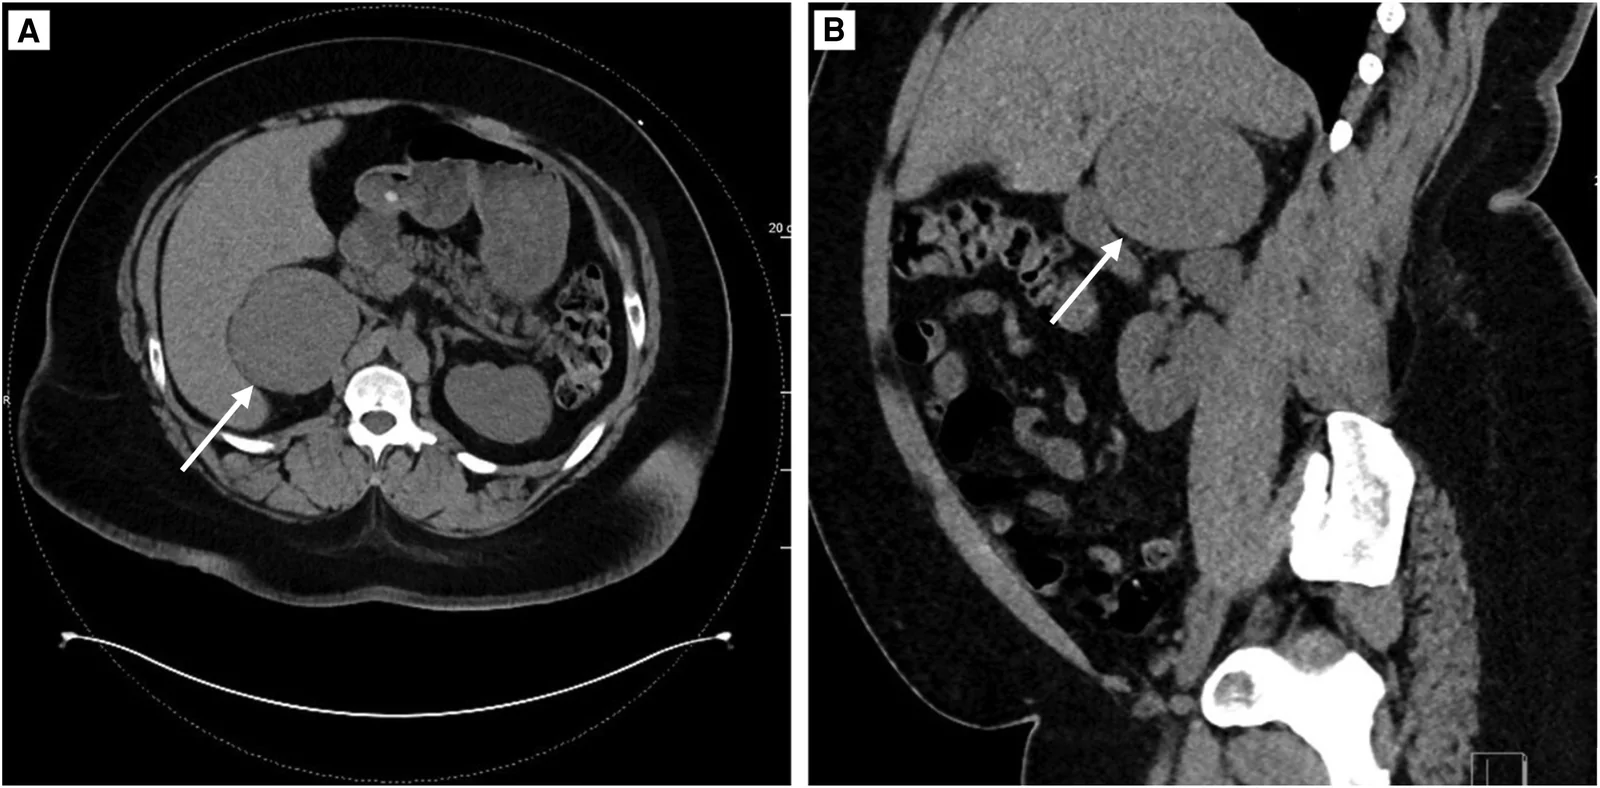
Noncontrast computed tomography of the abdomen showing the right adrenal mass in axial (A) and sagittal (B) planes. The lesion measured 8.4 × 8.3 × 7.9 cm and had enlarged from approximately 3 cm on a chest CT obtained in 2020, approximately 5 years before the current examination. White arrows indicate the adrenal mass.
